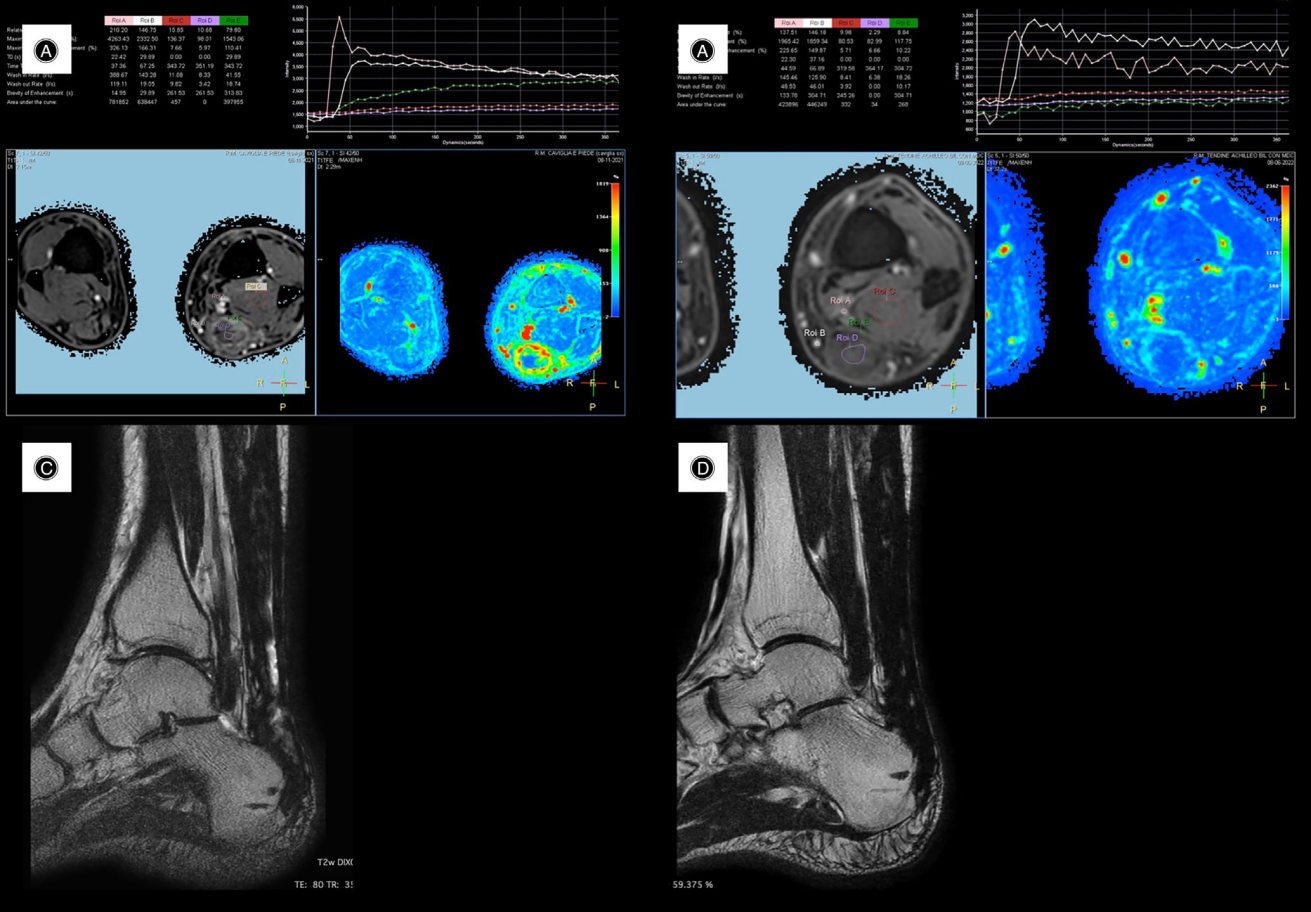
(A) Dynamic‐perfusion MRI at 12 months after surgical treatment. Incomplete and evolving tendon “regeneration.” Green‐red signal. (B) Dynamic‐perfusion MRI at 18 months after surgical treatment. Complete tendon “regeneration.” Blue signal. (C) Conventional MRI in sagittal T1 at 12 months after surgical treatment. Uneven signal of the tendon however continuous. (D) Conventional sagittal T1 MRI at 18 months after surgical treatment. Good restoration of tendon structure.
